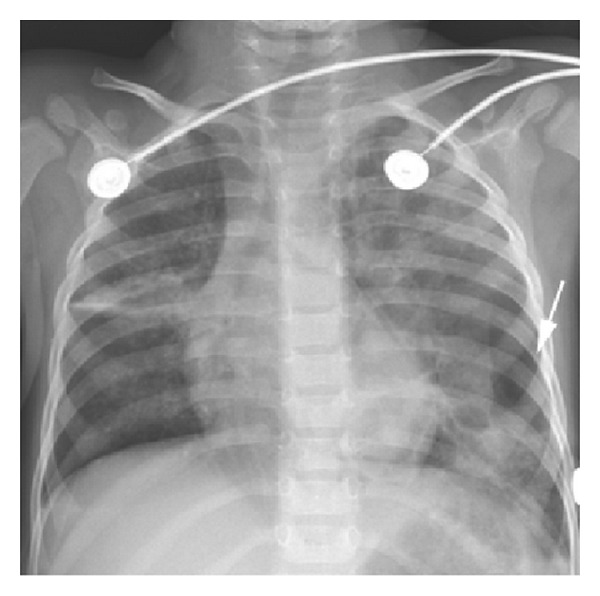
Chest X-ray showing large radiolucent and patchy cystic infiltration on the left lung field.
